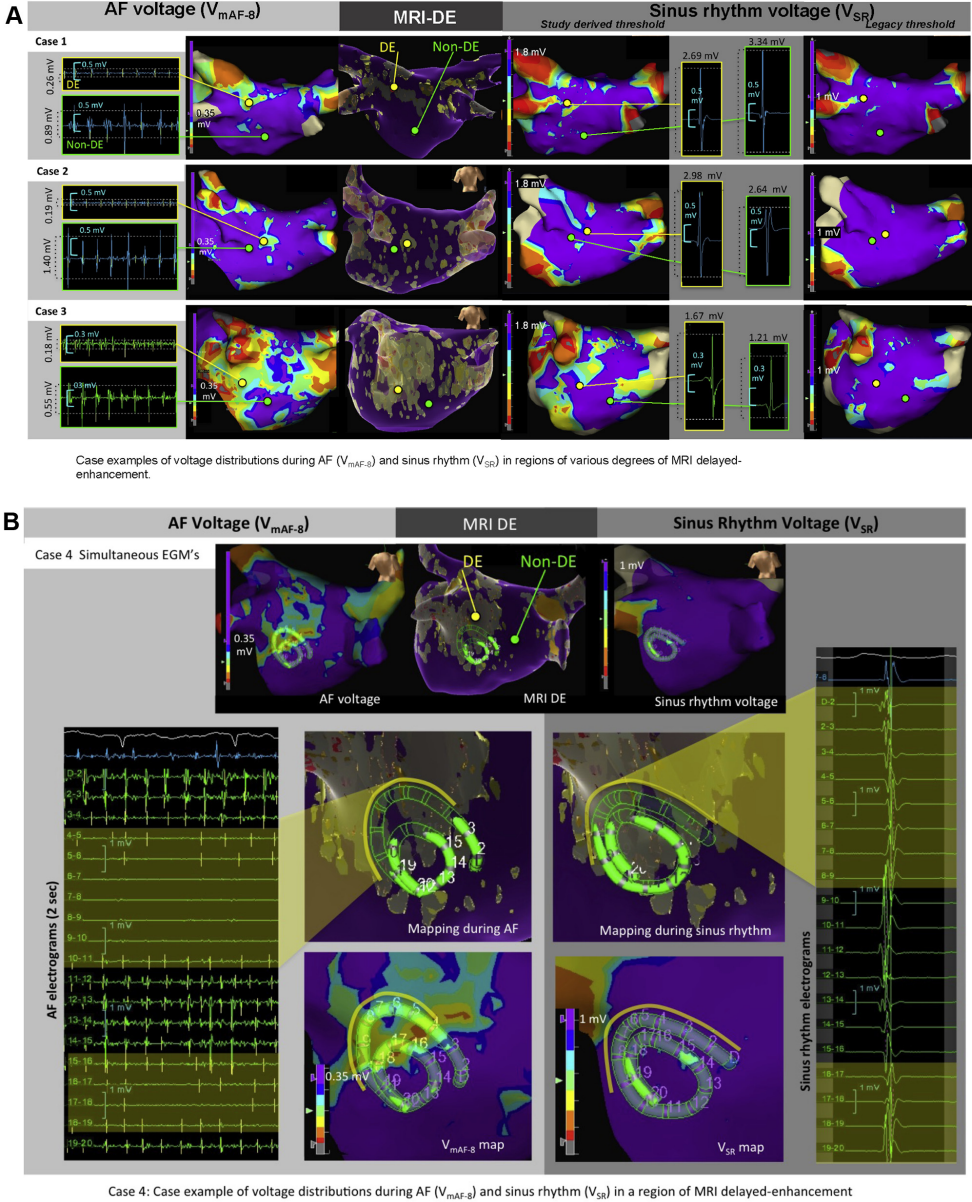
Case examples of atrial fibrillation (AF) and sinus rhythm (SR) voltage vs delayed-enhancement magnetic resonance imaging (MRI-DE). A: Case examples of AF (V_mAF-8_) and SR voltage with varying degrees of MRI-DE–detected fibrosis. In all cases, the AF voltage map (left) (cutoff range *0.05–0.35 mV*) provides a visual spatial match with MRI-DE (>2 SD M_BP_). SR voltage maps set to the legacy cutoff range (0.1–1.0 mV; right) lack the sensitivity to detect MRI-DE. As the V_SR_ threshold is increased to the study-derived threshold (1.8 mV; middle), sensitivity for MRI-DE detection is improved, whereas specificity is reduced. Representative electrograms are displayed from both DE/non-DE regions of each map. B: Case example with simultaneous electrograms sampled across the DE/non-DE border during both AF (left) and SR (right). During AF, the transition from non-DE to DE can be seen in both the differential amplitude of electrograms and in the V_mAF-8_ map (cutoff range *0.05–0.35 mV*). In contrast, electrograms during SR reveal no obvious differential. The V_SR_ map (cutoff range 0.1–1.0 mV) has no low-voltage zones.
